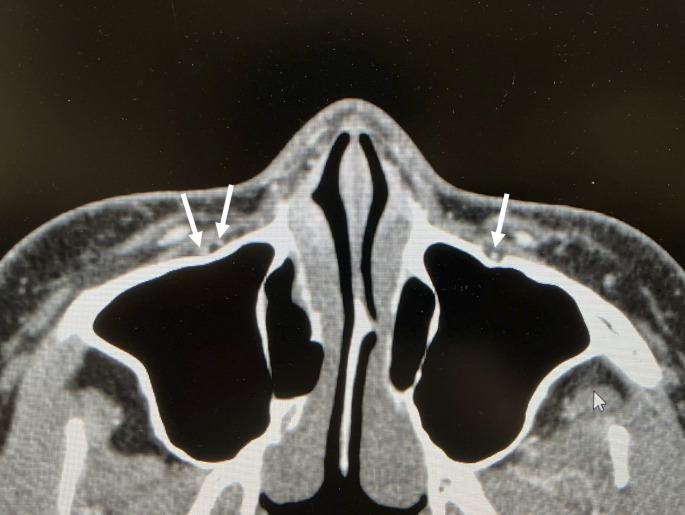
Axial contrast-enhanced CT image from a patient demonstrating the infraorbital vessels (arrows)
